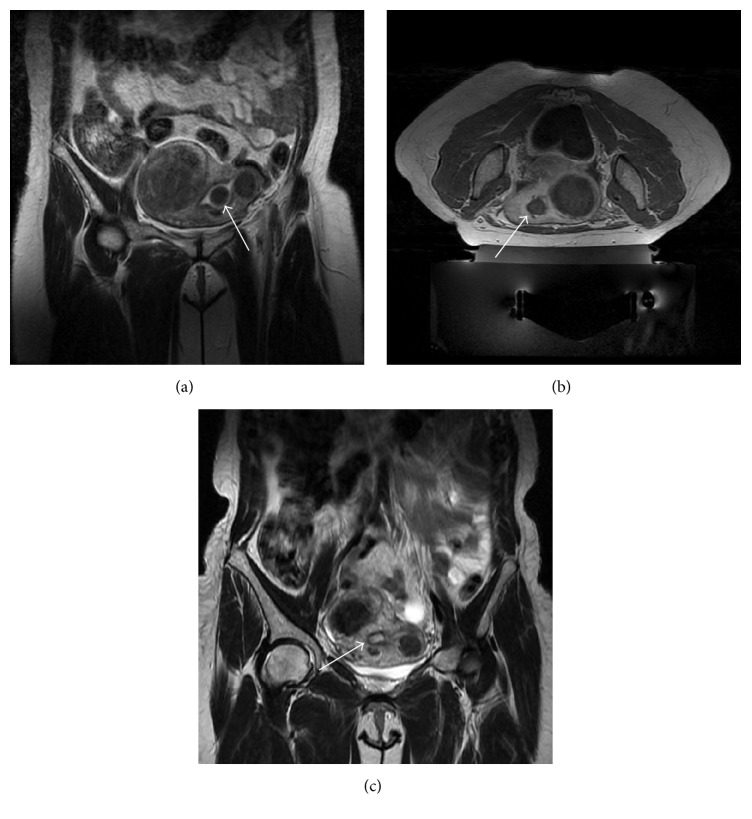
Case 3: (a) screening coronal T2-w showing the bigger intramural fibroid on the right wall of the uterus, the small intracavitary submucosal fibroid on the mid left (white arrow), and one more small intramural fibroid on the left wall of the uterus; (b) axial T1-w acquired after intravenous injection of paramagnetic contrast medium acquired at the end of the treatment showing treated fibroids as nonenhancing round lesions (white arrow on the small intracavitary submucosal fibroid); (c) in a follow-up MRI, the small intracavitary submucosal fibroid was no more appreciable; the white arrow shows the “empty” uterine cavity after spontaneous vaginal expulsion.
